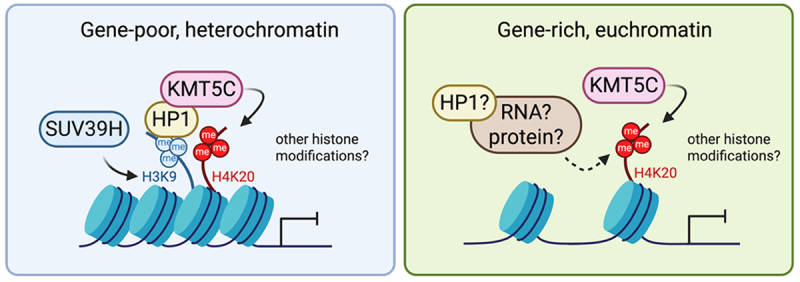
Sequential deposition of H4K20me3 by KMT5C in gene-poor, heterochromatin: HP1 binds to H3K9me3 (catalyzed by SUV39H) and recruits KMT5C to chromatin. KMT5C utilizes H4K20me1, which was established by KMT5A, and generates H4K20me3. It has been suggested that HP1-inpendendent KMT5C recruitment pathways exist in gene-rich euchromatin, but this model requires further characterization. It would also be essential to investigate how H4K20me3 engages in crosstalk with other histone modifications to orchestrate the effect of H4K20me3.
